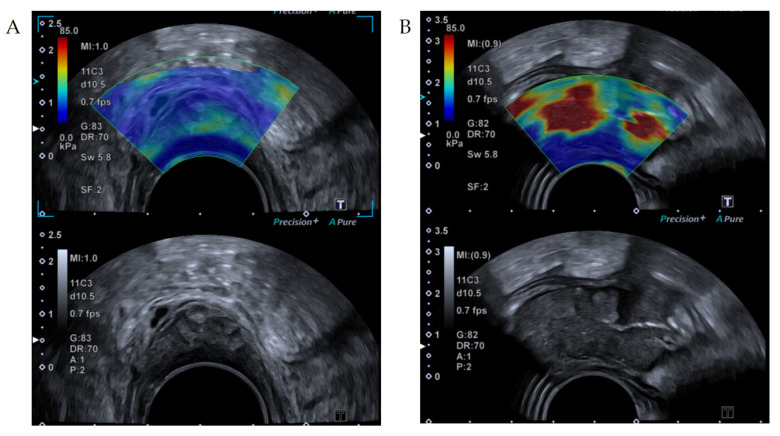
Shear wave elastography images of an (A) adenoma and a (B) adenocarcinoma. Upper image shows the elastogram superimposed with a B-mode image. Bottom image is the corresponding B-mode ultrasound image allowing the examiner to place the region of interest within the tumor area. (A): The elastogram shows soft values (blue and green colors). (B): Adenocarcinoma with high elastography values (red colors).
